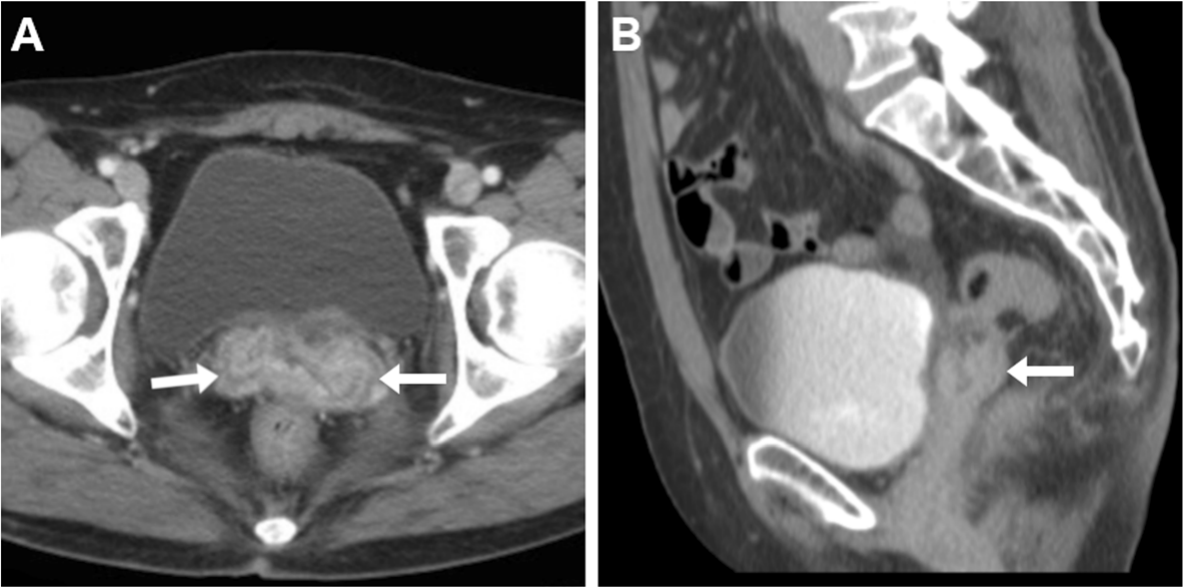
Differential diagnosis of VF-related vaginitis: expected CM-enhanced CT appearance in axial (a) and sagittal (b) planes after recent vaginal hysterectomy with a “swollen” configuration in transverse planes, circumferential oedematous mural thickening and marked mucosal enhancement
